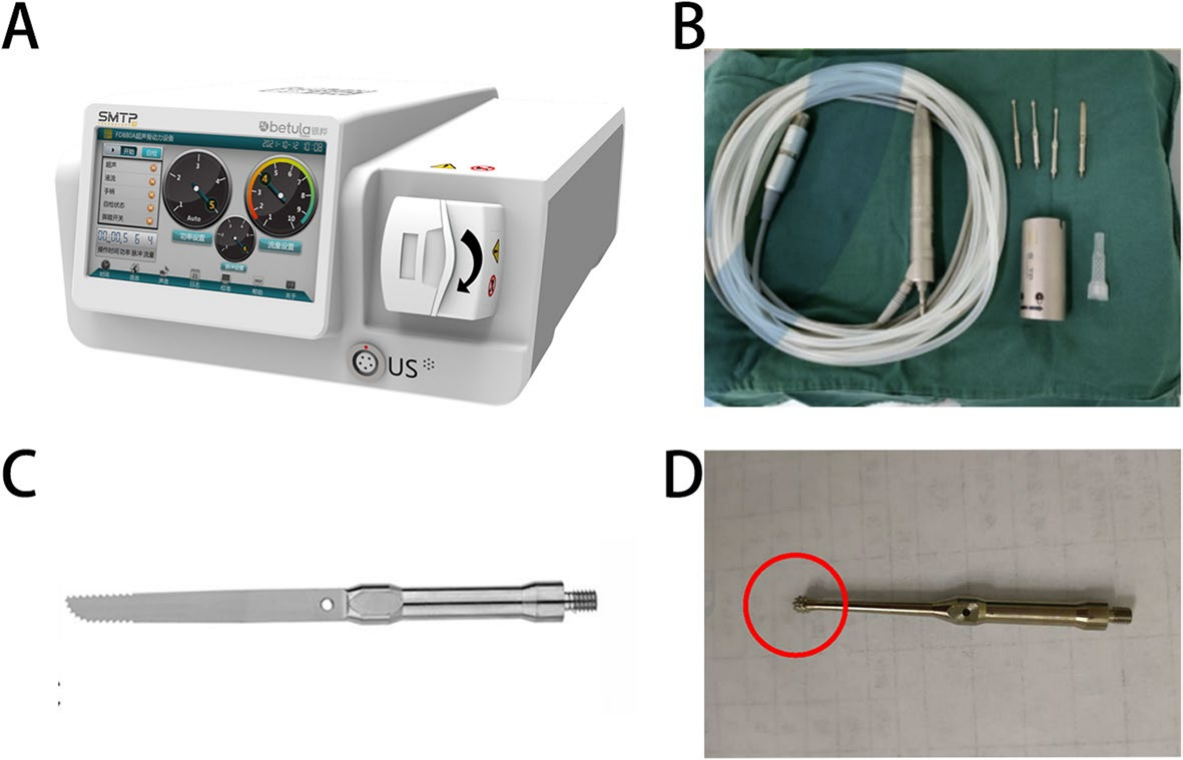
Introduction of Ultrasonic Bone curette Instrument System. A Ultrasonic bone dynamic system XD880A. B Handle and various types of knife head. C Straight blade head. D spherical knife head
